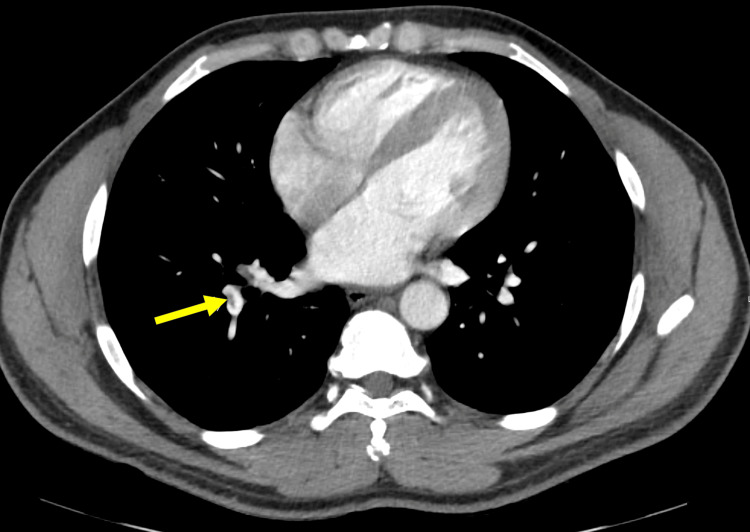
Pulmonary embolism on CT angiogram of the chest (arrow).
